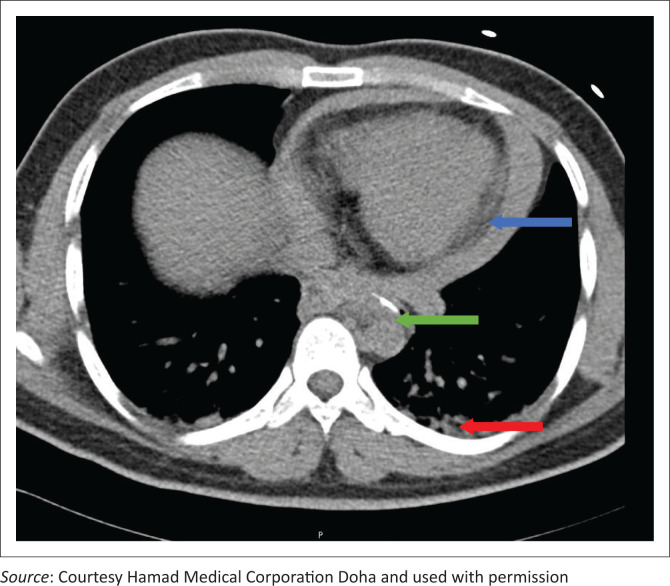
Computed tomography scan showing pericardial effusion (blue arrow), hook-shaped radio-opaque shadow (green arrow) and blood in the thorax (red arrow).
